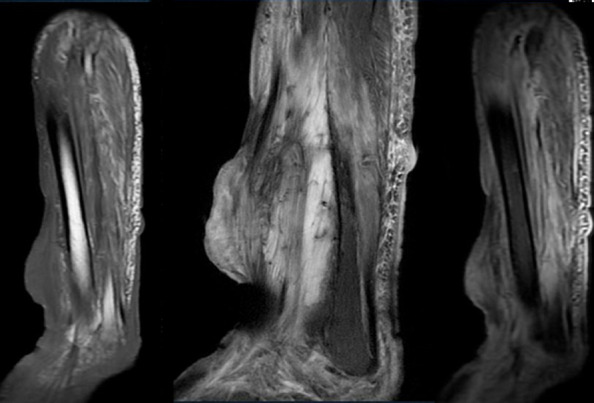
MRI of the same patient (sagittal T1 and T2 and STIR images) shows a T1 iso to hypo intense lesion within the skeletal muscle; T2 hyperintense edema is noted; no bone marrow involvement is seen
